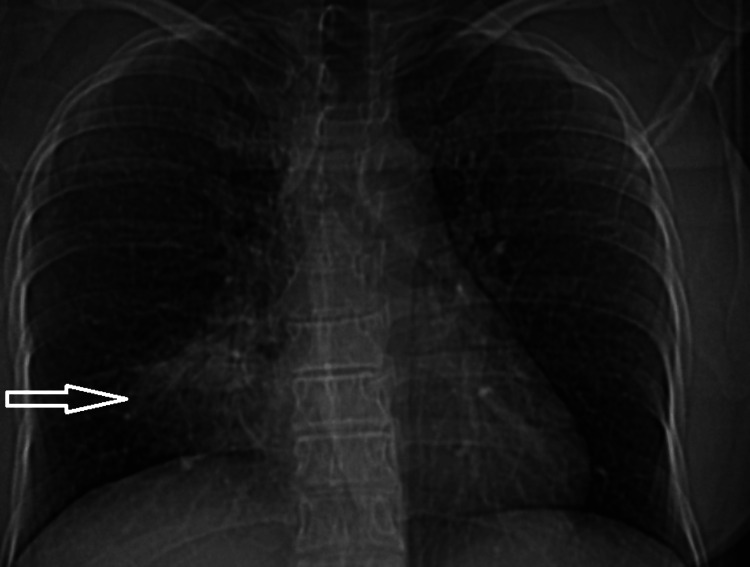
A right paracardiac pneumonic infiltration is marked with an arrow on the plain chest radiograph.
